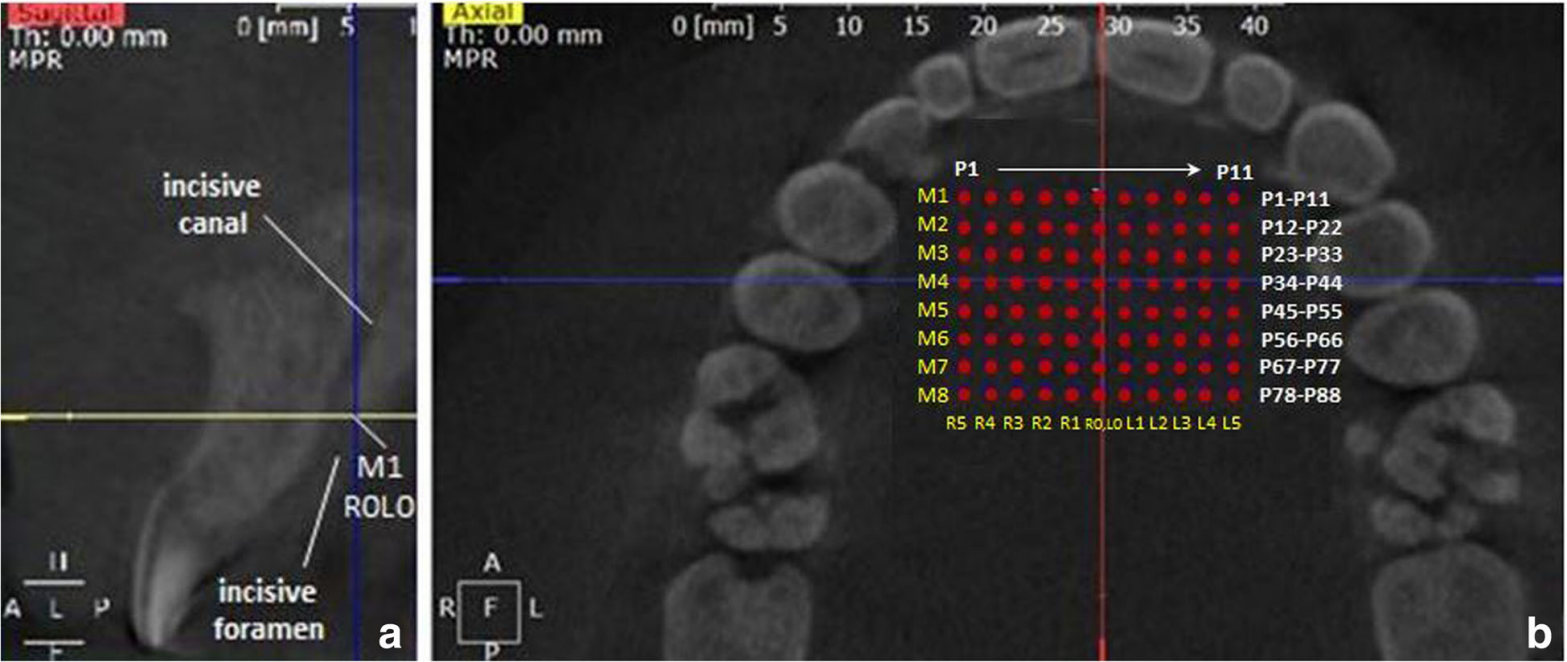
a Sagittal CBCT window depicting the alignment of the axial (yellow line) and coronal (blue line) planes for measurements. The crossing point in the median-sagittal plane denotes the location of the first palatal height measurement (M1, R0L0). b Axial CBCT window depicting the aligment of the sagittal plane (red line) for palatal bone height measurements, carried out according to a measurement grid (red dots), constructed starting from the posterior-cranial osseous border of the incisive foramen (M1, R0L0)
